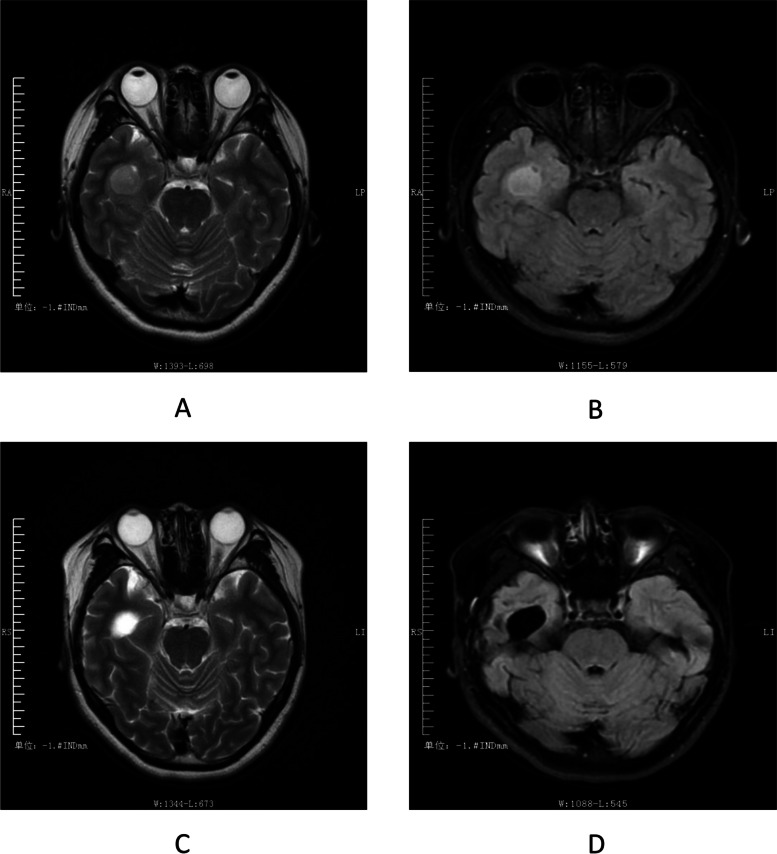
MRI image of the patient (Example 2). T2W1 was a slightly high signal (a), and Flair was a high signal (b). Postoperative re-examination showed that T2W1 was a high signal (c) and Flair was a low signal (d)
